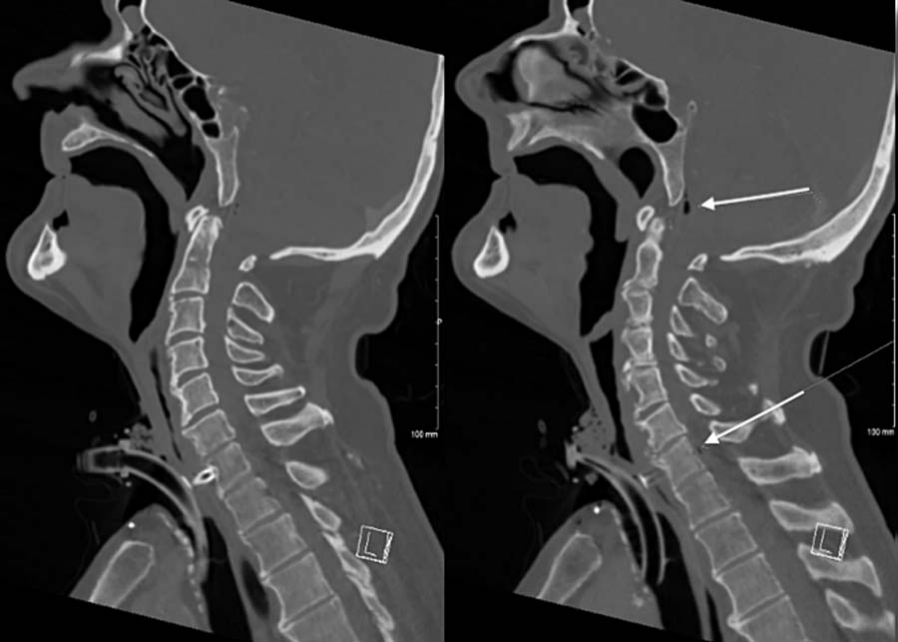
CT Neck demonstrating erosion of TEP into the C7 cervical resulting in osteomyelitis and a gas containing anterior epidural collection.
